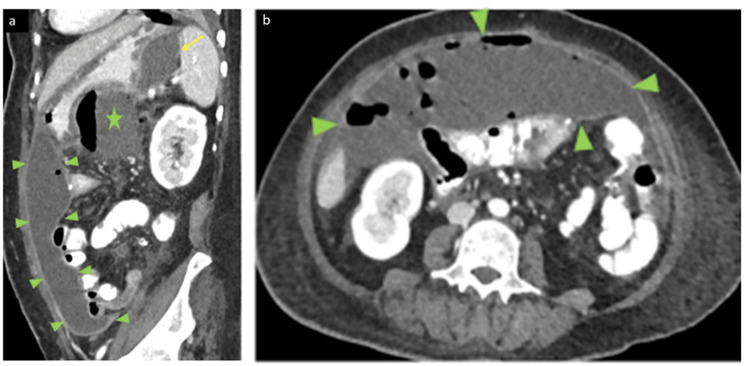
Postoperative contrasted abdominal CT scan Sagittal (a) and Axial (b) images show huge anterior abdominal peripherally enhancing fluid collection with gas bubble underlying the anterior abdominal wall (arrow heads) displacing the bowel posteriorly. Another two smaller collections also were noted along the gastro-splenic space (yellow arrow) and inferior to the stomach (star) abutting its greater curvature with the latter shows air fluid level.
